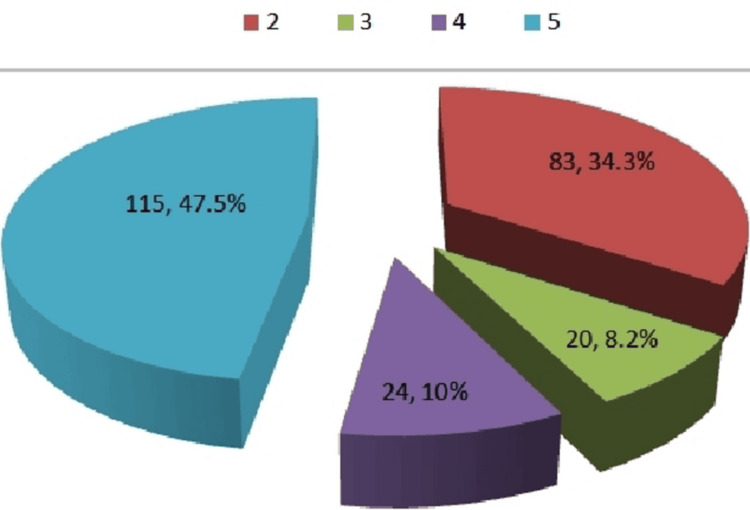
Frequency of different lesions on HR US categorized on BI-RADS HR US: high-resolution ultrasound; BI-RADS: Breast Imaging Reporting and Data System
